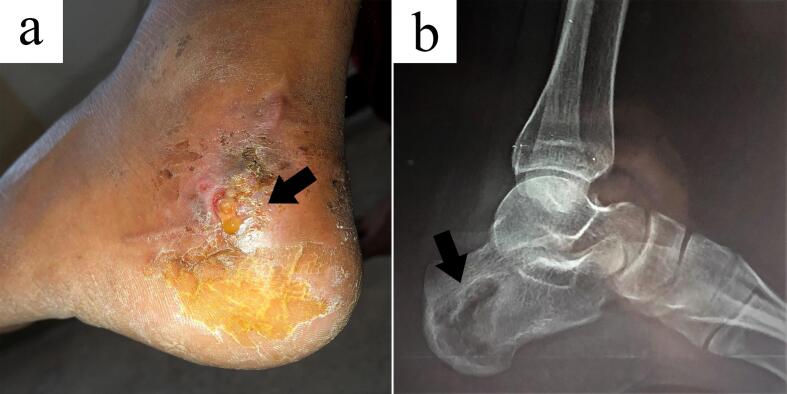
Figures showing postoperative recurrence of the disease. (A) Draining sinus seen on the posteroinferolateral aspect of the left ankle (Arrow). (B) Enlarged osteolytic lesion seen in lateral radiograph of the left foot in the same region (B).
